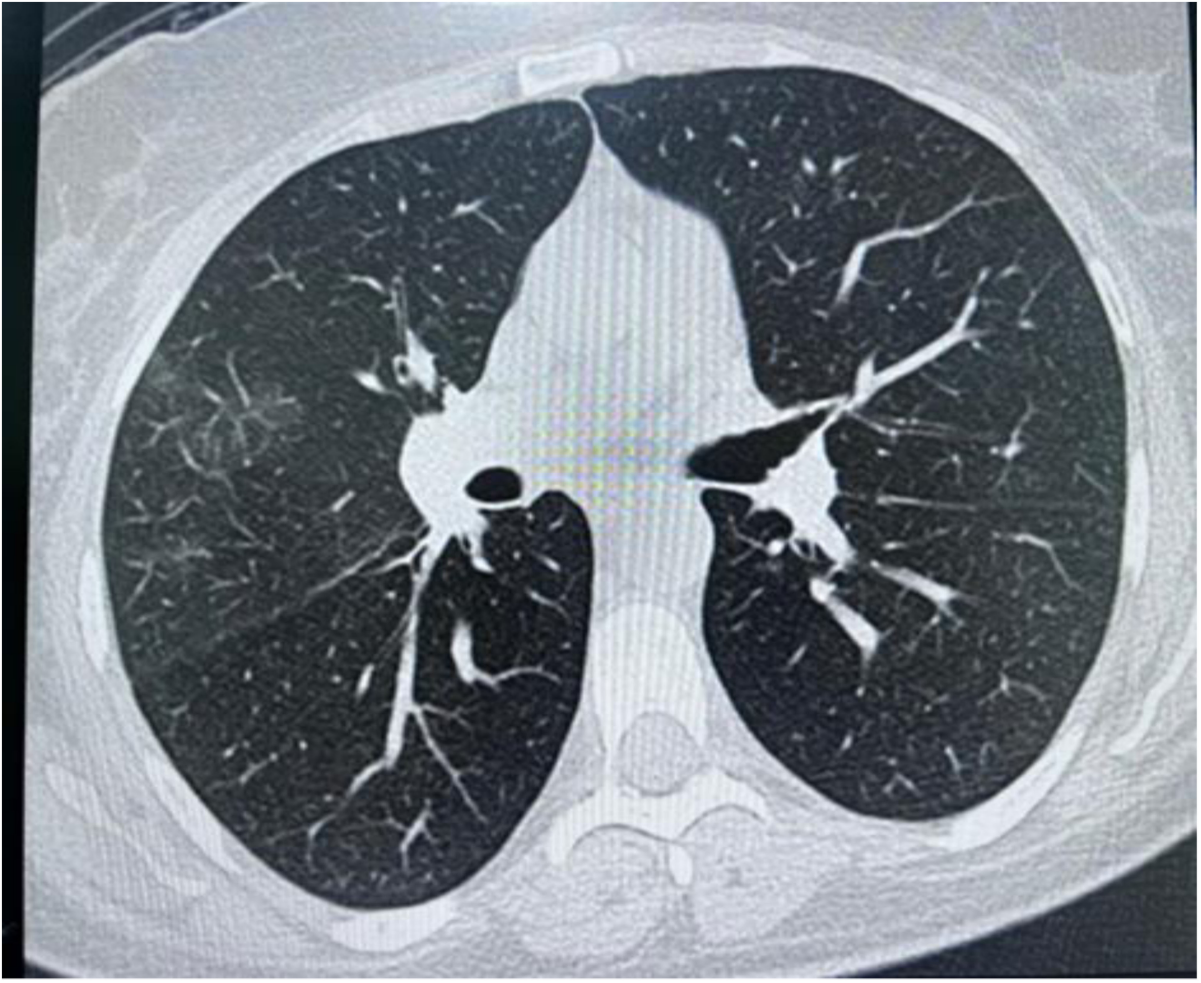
High resolution computed tomography (HRCT) chest showing peripheral and central faint patchy areas of ground glass densities within the lower segment of right upper lobe.
